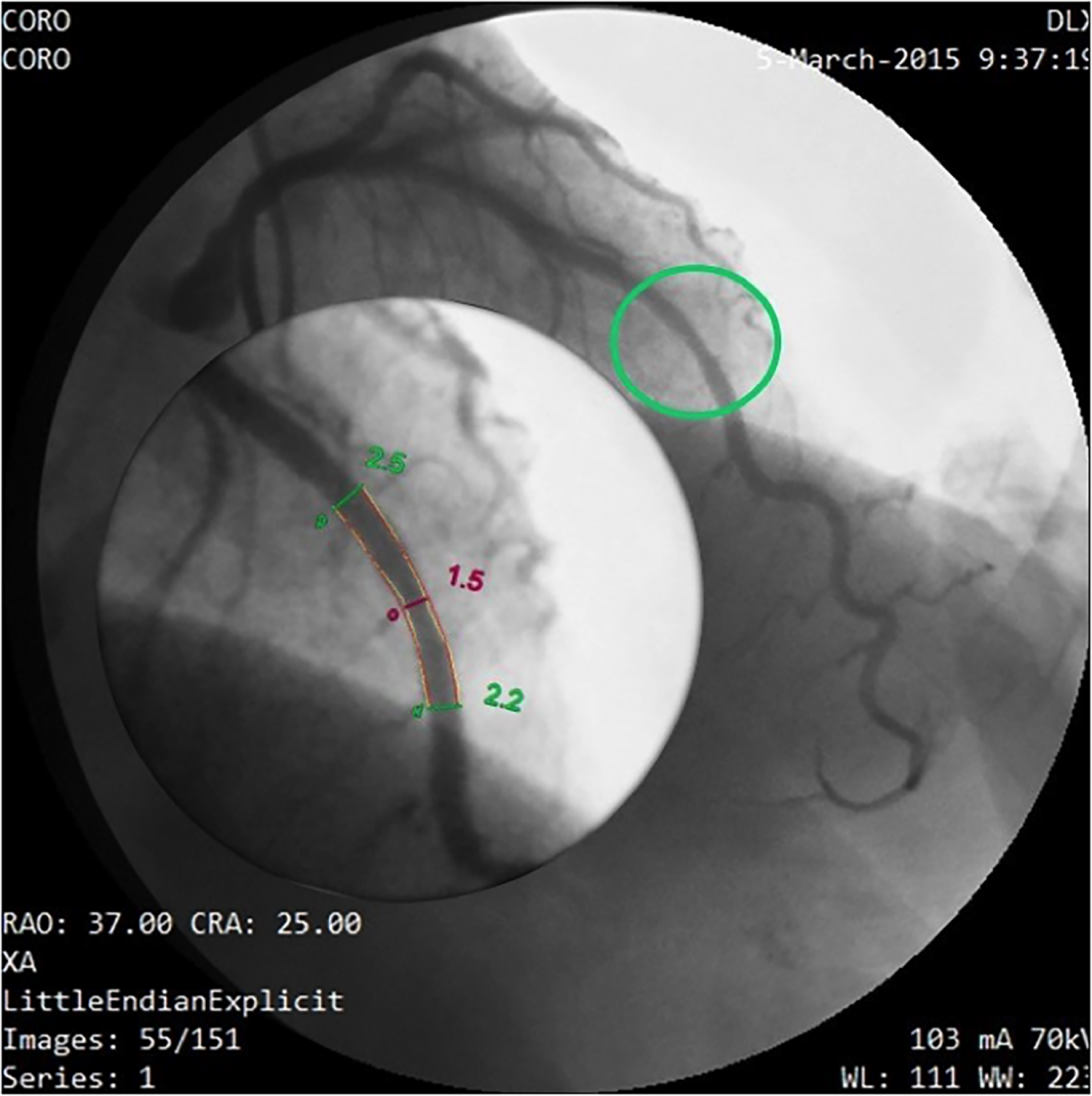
Coronary angiography in the same patient as in Figures 10, 11. Same layout as in the previous angiographic image. In this cranial right oblique view, the site of the mid LAD mild stenosis (encircled in green) is depicted and quantified (40% CSA stenosis) that confirms the E-Doppler TTE results in terms of location ad severity. In addition, angiography shows LAD luminal irregularities and subocclusive stenosis of the right coronary artery (not shown) so clearly pointing out a diffuse atherosclerosis. However, no deduction can be done of the real functional impact of this apparently mild athero. QCA, quantitative coronary angiography; LAD, left anterior descending coronary artery; CSA, cross sectional area.
